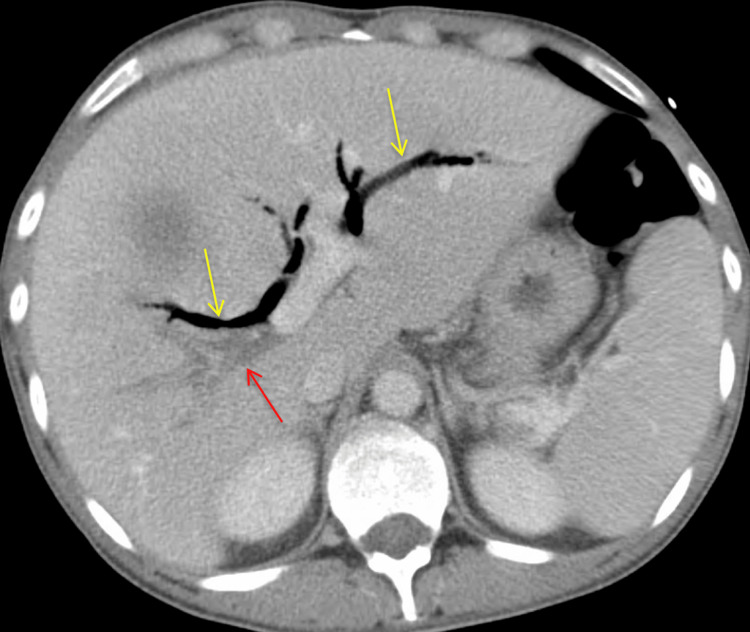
Additional view of CT abdomen and pelvis with IV contrast (axial view). The image shows the septic thrombosis of the right portal venous system (red arrow) with mildly distended intrahepatic biliary ducts and air in the biliary tree (yellow arrows).
